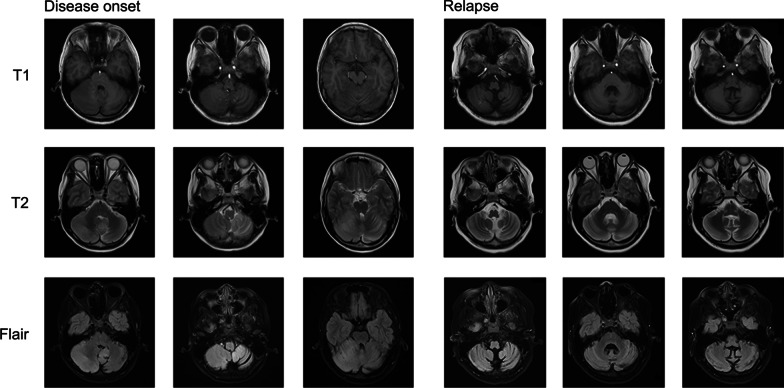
Cranial MRI images of the case in the phases of disease onset and relapse, including T1, T2, and FLAIR sequences
